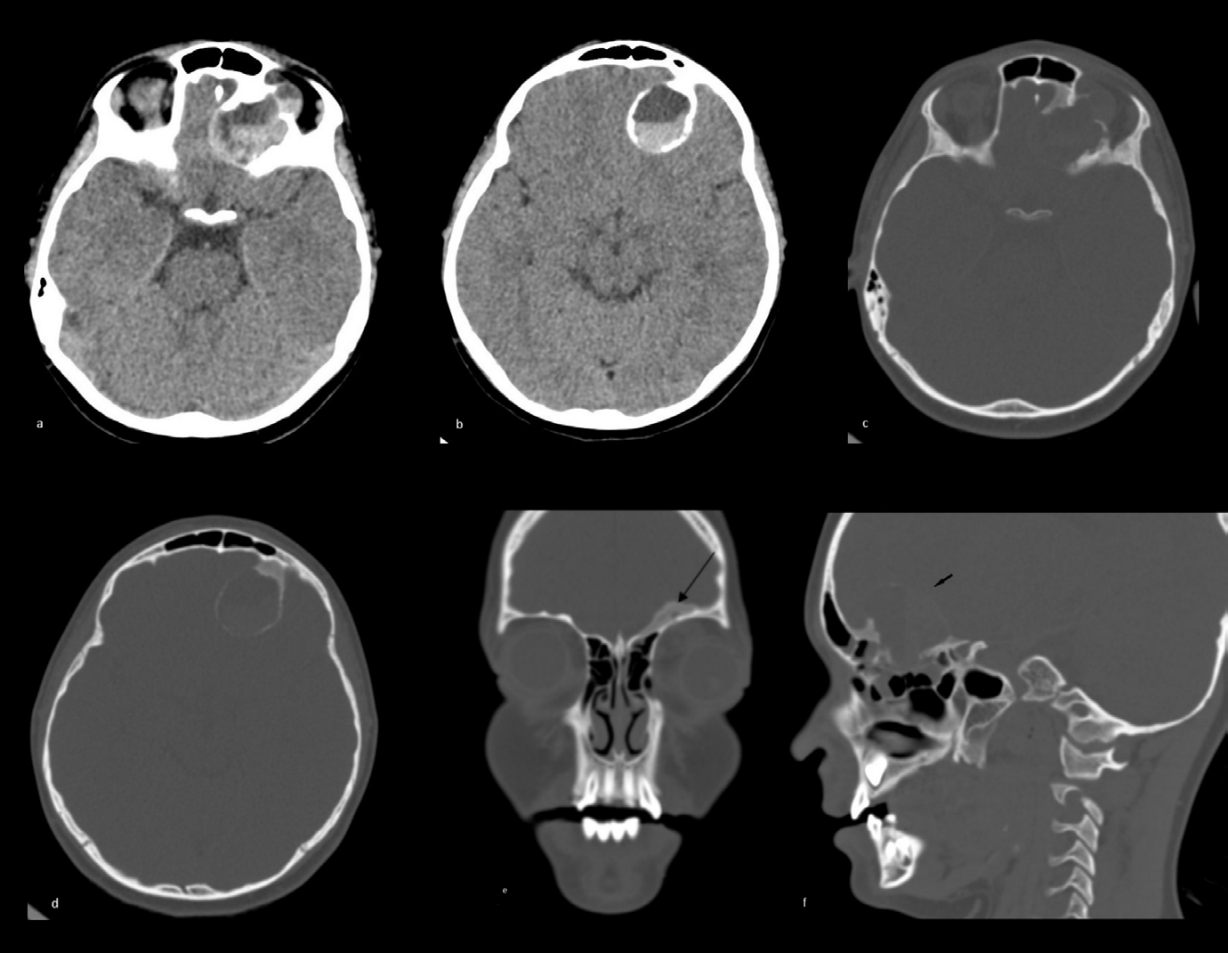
CT head: Soft tissue window (a, b) showing a large expanding space occupying lesion involving the left ethmoid air cells with destruction of the left orbital roof and left orbital and left inferior frontal intracranial extension. High-density fluid level indicating blood within the lesion (haematocrit effect). Bone window (c, d, e, f) showing calcified rim of the cystic lesion and ground-glass density (thick black arrow) There is a bone defect of the left cribriform plate allowing extension of the lesion intra cranially forming a large extra-axial component with a calcified rim extending under the left frontal lobe (short black arrow).
